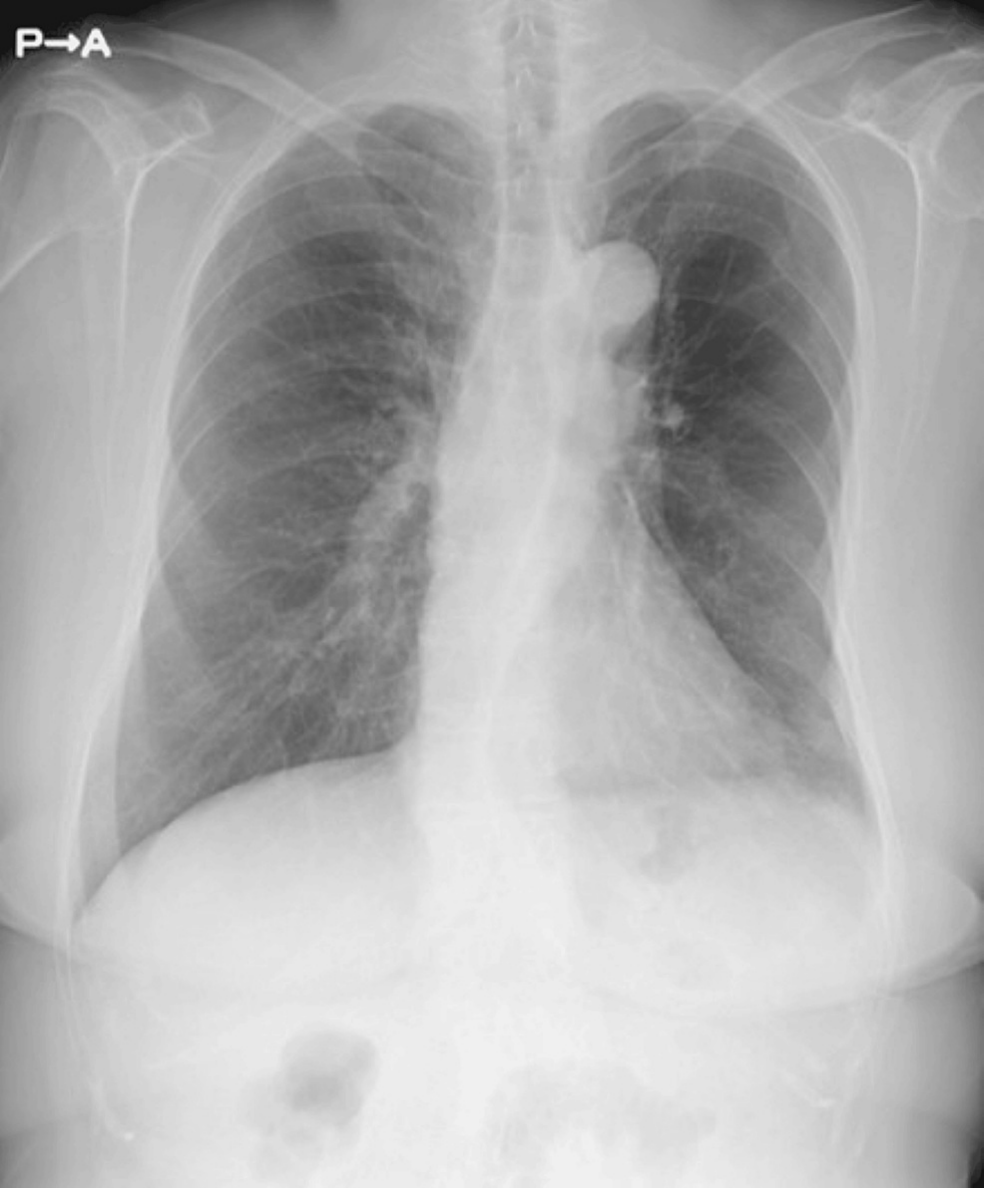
A chest radiography finding after discharge. The left diaphragm appeared smooth.
